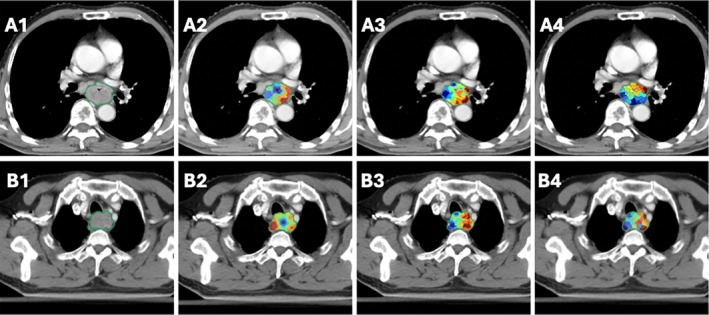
(A) A 66‐year‐old female patient with esophageal squamous cell carcinoma. The patient received IMRT at a total dose of 50 Gy and developed recurrence 15 months later. (A1) Arterial‐phase CT with lesion delineation. (A2) Nested habitat analysis results. (A3) Intensity‐based k‐means clustering results. (A4) Super pixel clustering results. (B) A 75‐year‐old male patient with esophageal squamous cell carcinoma. The patient received IMRT at a total dose of 50 Gy and developed recurrence 9 months later. (B1) Arterial‐phase CT with lesion delineation. (B2) Nested habitat analysis results. (B3) Intensity‐based k‐means clustering results. (B4) Super pixel clustering results.
